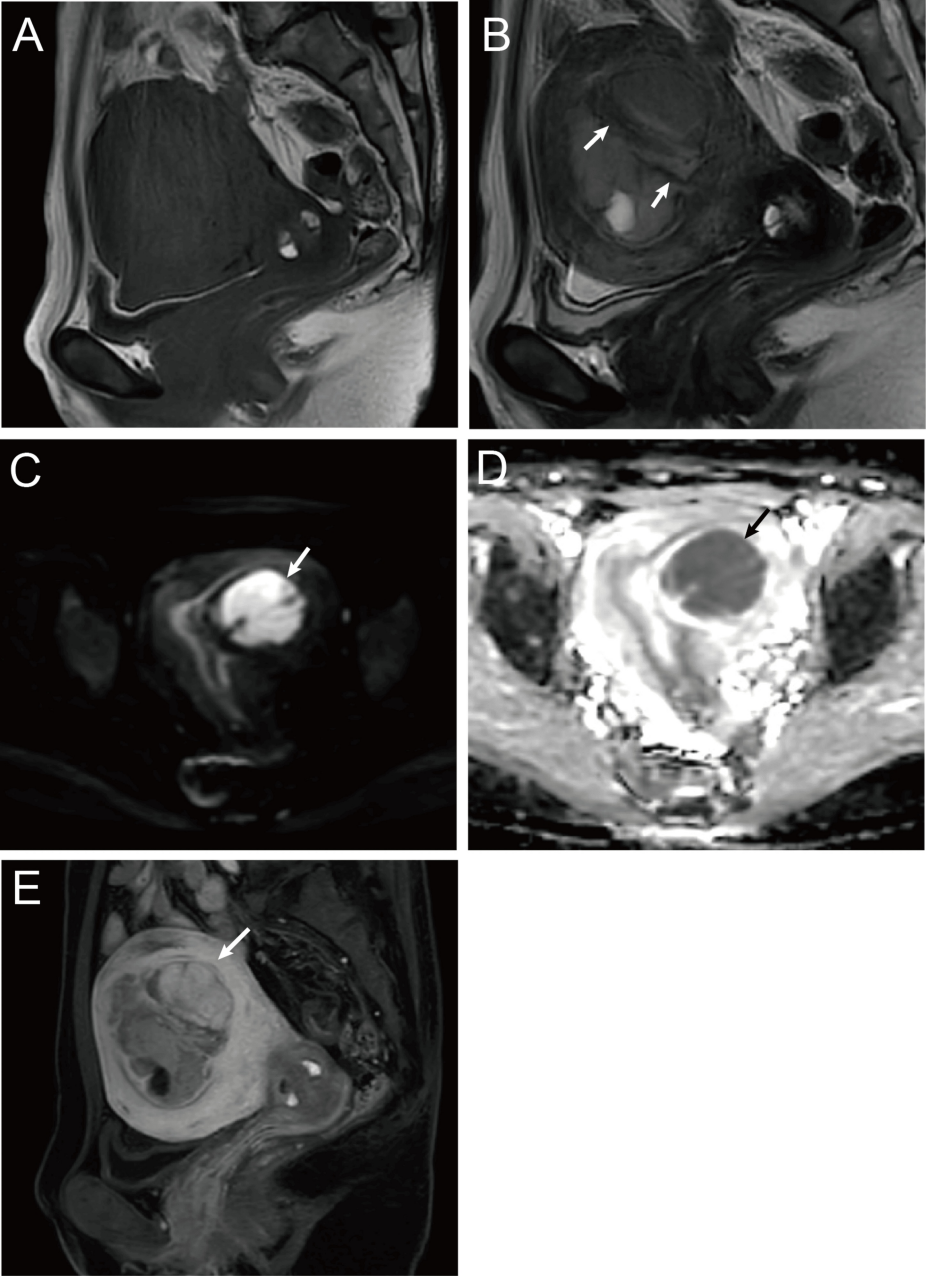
Fifty-two-year-old woman with LGESS (case 6) A mass was found in the uterine wall showing isointensity on T1WI (A) and heterogeneous hyperintensity with hypointense bands (arrows) on T2WI (B). Diffusion-weighted imaging (DWI) (C) and apparent diffusion coefficient (ADC) map (D) showing hyperintense and hypointense masses, respectively (arrows). Sagittal contrast-enhanced T1WI with fat suppression (E) showed a heterogeneous enhanced mass. T1WI: T1-weighted imaging; T2WI: T2-weighted imaging; LGESS: low-grade endometrial stromal sarcoma
